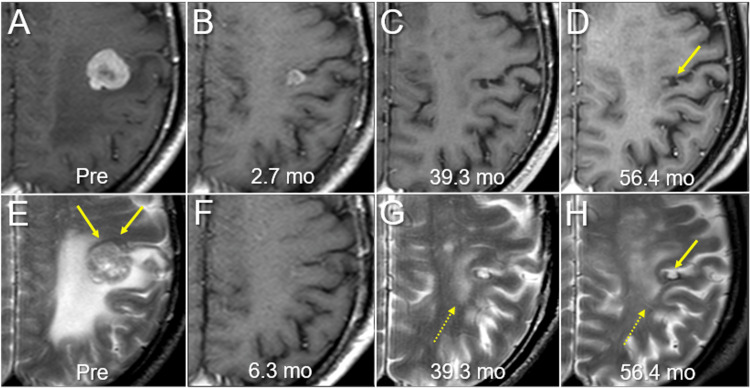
Magnetic resonance images before and after radiosurgery for brain metastasis. The images show axial CE-T1-WI (A-D, F); axial T2-WI (E, G, H); 12 days before SRS (Pre) (A, E); at 2.7 months (mo) after SRS (B); at 6.3 months (F); at 39.3 months (C, G); and at 56.4 months (D, H). (B, F) T2-WI at 2.7 and 6.3 months after SRS were not acquired. All images were co-registered and are shown in the same magnification and coordinates. (E) The iso-intensity brain structure (arrows in F), probably the cerebral cortex, is distinguishable from the BM with heterogeneous high intensity. (B) Remarkable tumor shrinkage along with almost disappearance of the peritumoral edema is observed at 2.7 months. (F) Almost complete remission is confirmed at 6.3 months. (C, D, G, H) At 39.3 and 56.4 months, the tumor remnant (arrows in D, H) is visible as a cavitary lesion surrounded by a low-intensity rim on T2-WI with not the whole circumference, just bordering the central sulcus at the ventral side. No abnormal intensity is observed in the surrounding iso-intensity cerebral cortex, although high-intensity changes are observed in the surrounding deep white matter (dashed arrows in G, H). CE: contrast-enhanced; WI: weighted image; SRS: stereotactic radiosurgery; BM: brain metastasis
